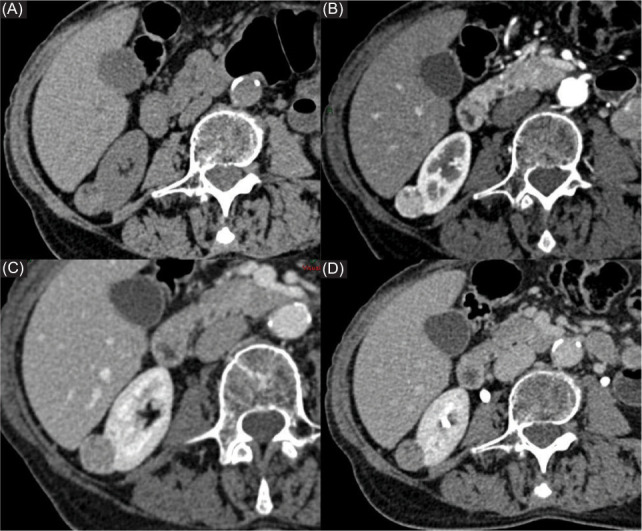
Contrast-enhanced CT scan revealed a 3.0×2.7-cm oval mass in the upper pole of the right kidney with (A) heterogeneous density in based scan due to (B–D) sporadic fat foci and a progressive enhancement observed in the major part of the tumor.
